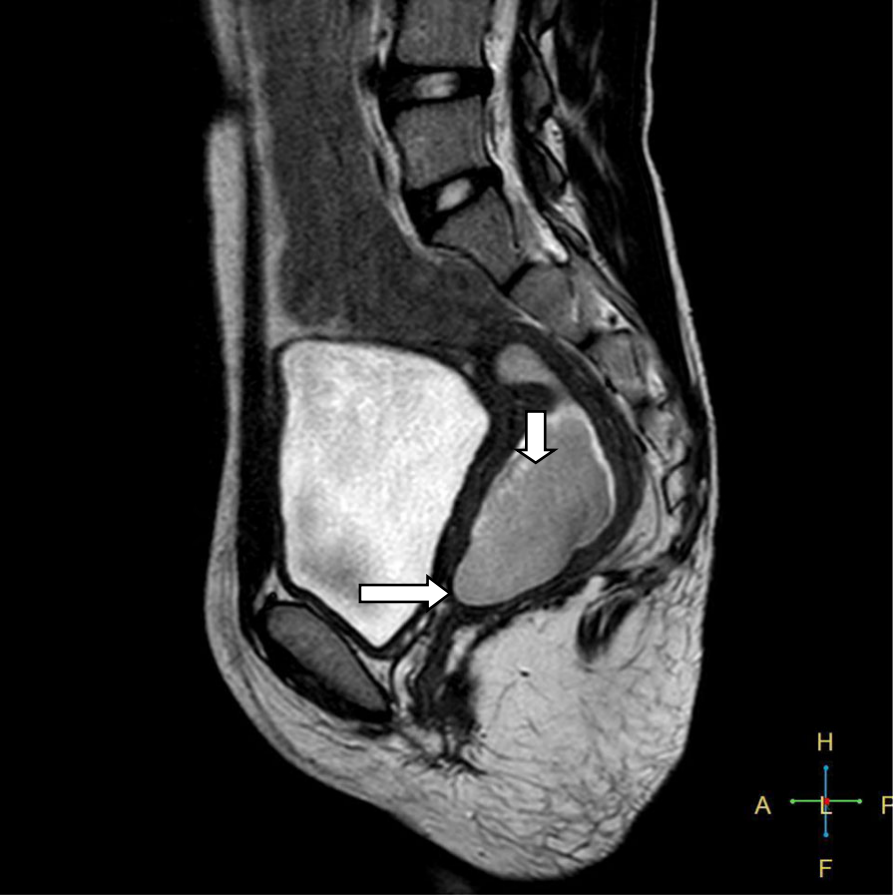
Sagittal T2-weighted image showing dilation of cervical canal (*short arrow*) with abrupt termination at vaginal fornix (*long arrow*), resulting in obstructed hemivagina. Endometrial cavity was mildly dilated and contained hemorrhagic collection
